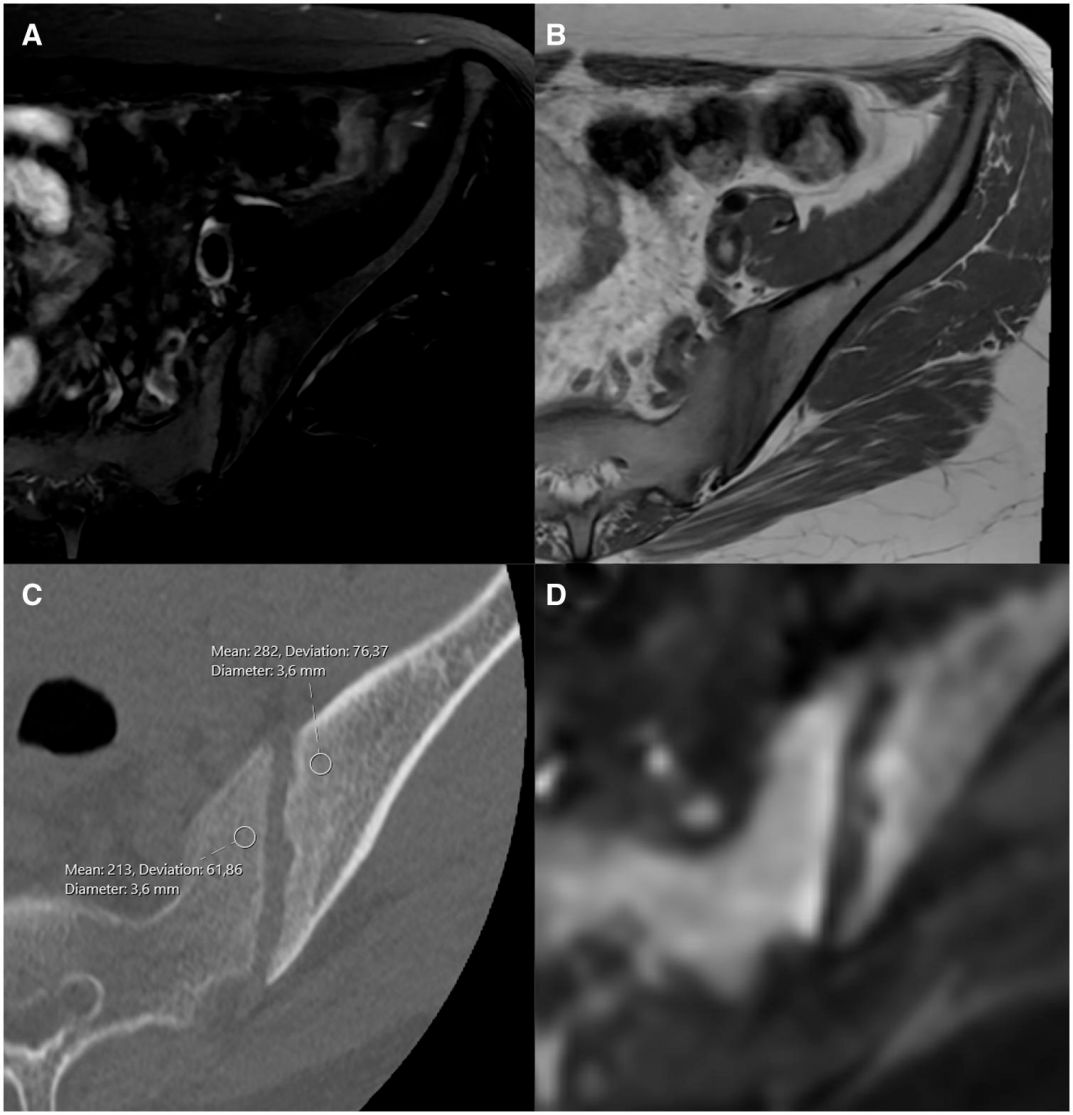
(A) Hyperintense signal on the fat-suppressed axial T2 MRI at the iliac and sacral facets of the left sacroiliac joint. (B) Hypointense signal on the axial T1 MRI at the iliac and sacral facets of the left sacroiliac joint. (C) On low-dose CT density measurements made through the ROI circle are taken in the subchondral areas of the sacral and iliac surfaces of the left sacroiliac joint. (D) Axial diffusion MRI shows signal enhancement in the subchondral area.
